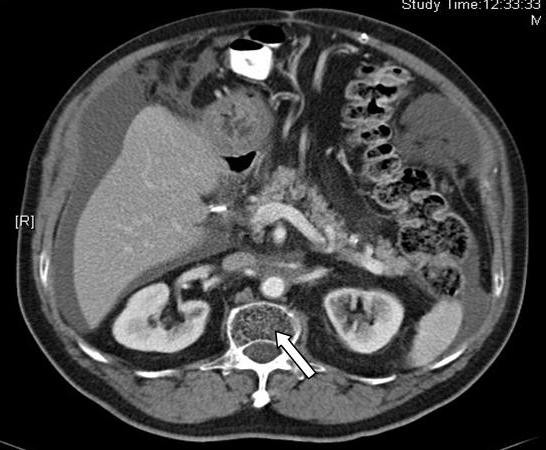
Post radiotherapy CT scan showing reduction in tumour (arrow) with ascites.
